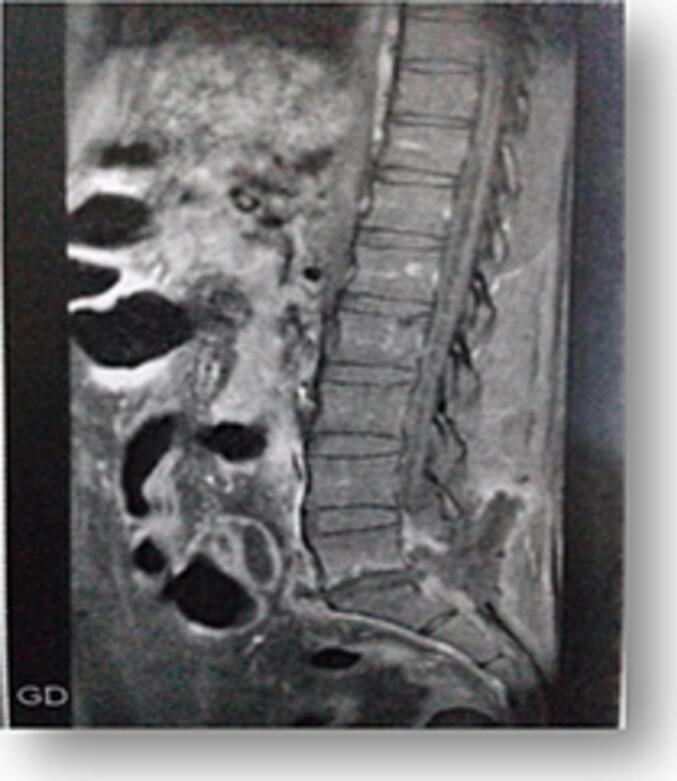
MRI showing lumbar wound collection.
